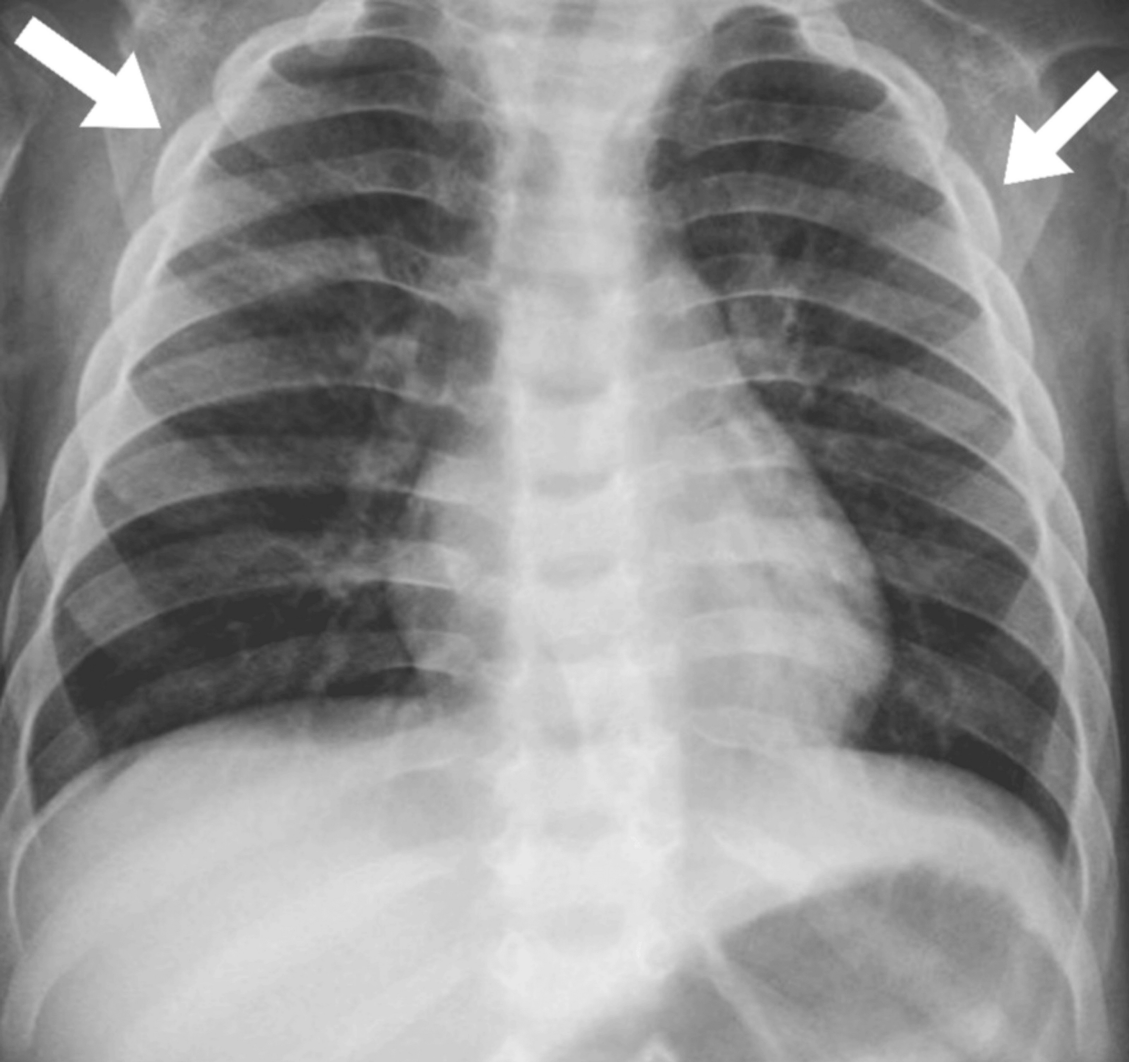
Chest X-ray displaying broad, “oar”-shaped ribs, as indicated by the arrows
